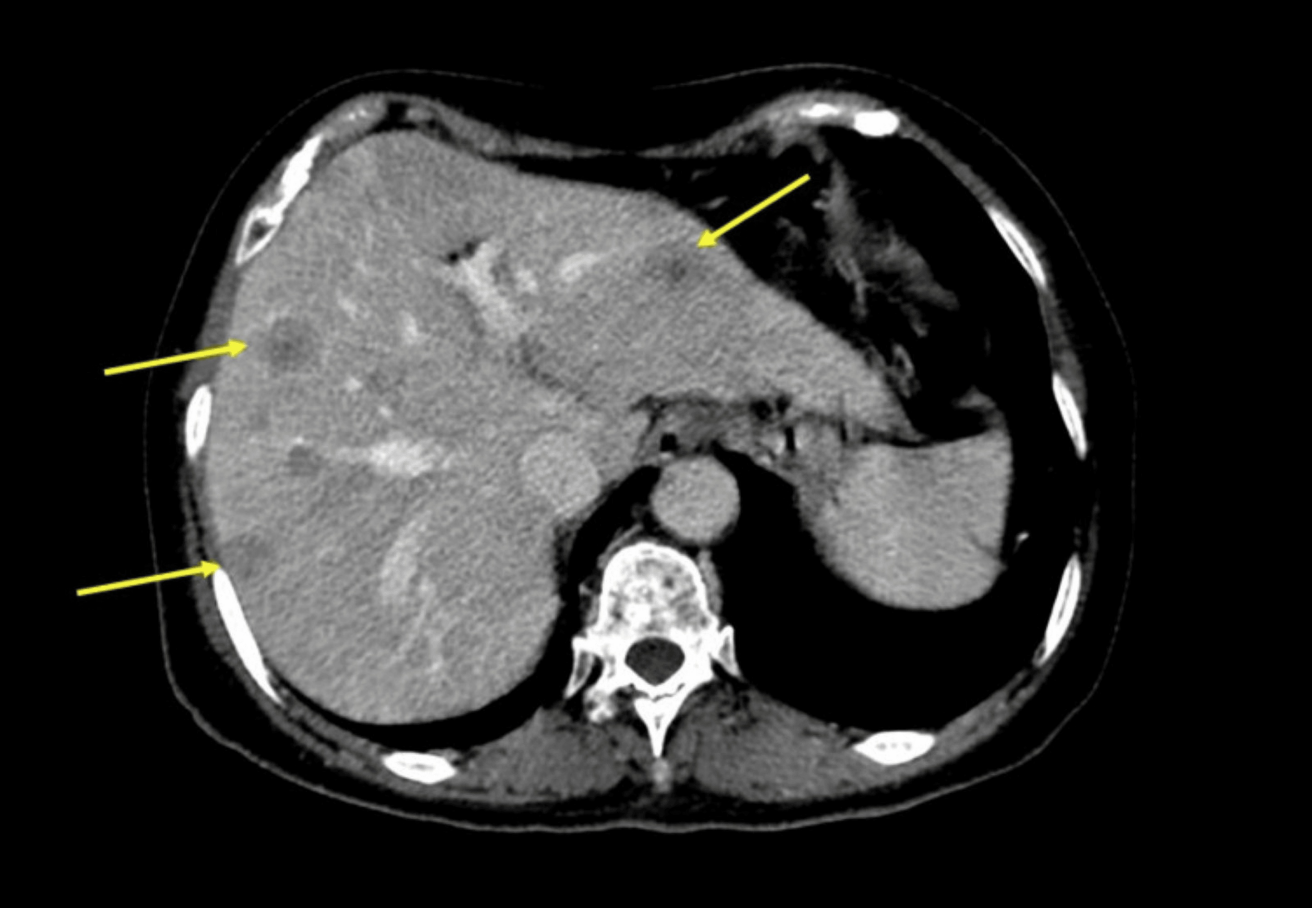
Contrast-enhanced abdominal CT scan showing multiple solid nodules in both hepatic lobes (yellow arrows) due to metastasis.
